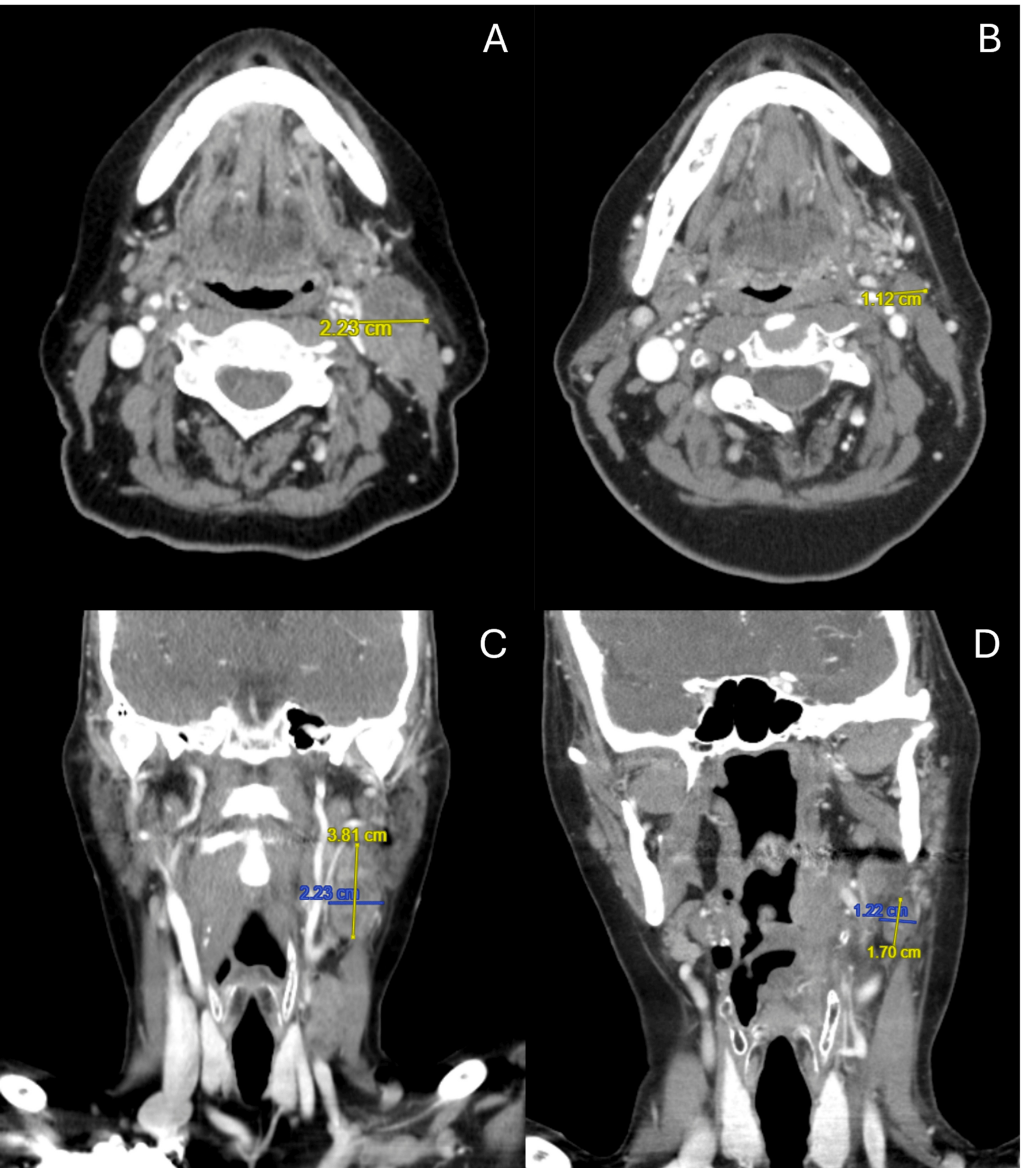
Diagnostic contrast-enhanced CT scan two months before and after SBRT Representative slices of the left level II lymph node in axial (A, B) and coronal (C, D) orientations. Panels A and C demonstrate the appearance and 2.2x3.8 cm size of the lymph node two months before SBRT. Panels B and D demonstrate the residual lymph node decreased in size to 1.2x1.7 cm two months after SBRT. There was a resolution of the treated level III lymph node in the two months after SBRT CT. The primary disease was initially CT occult. SBRT: Stereotactic body radiation therapy
